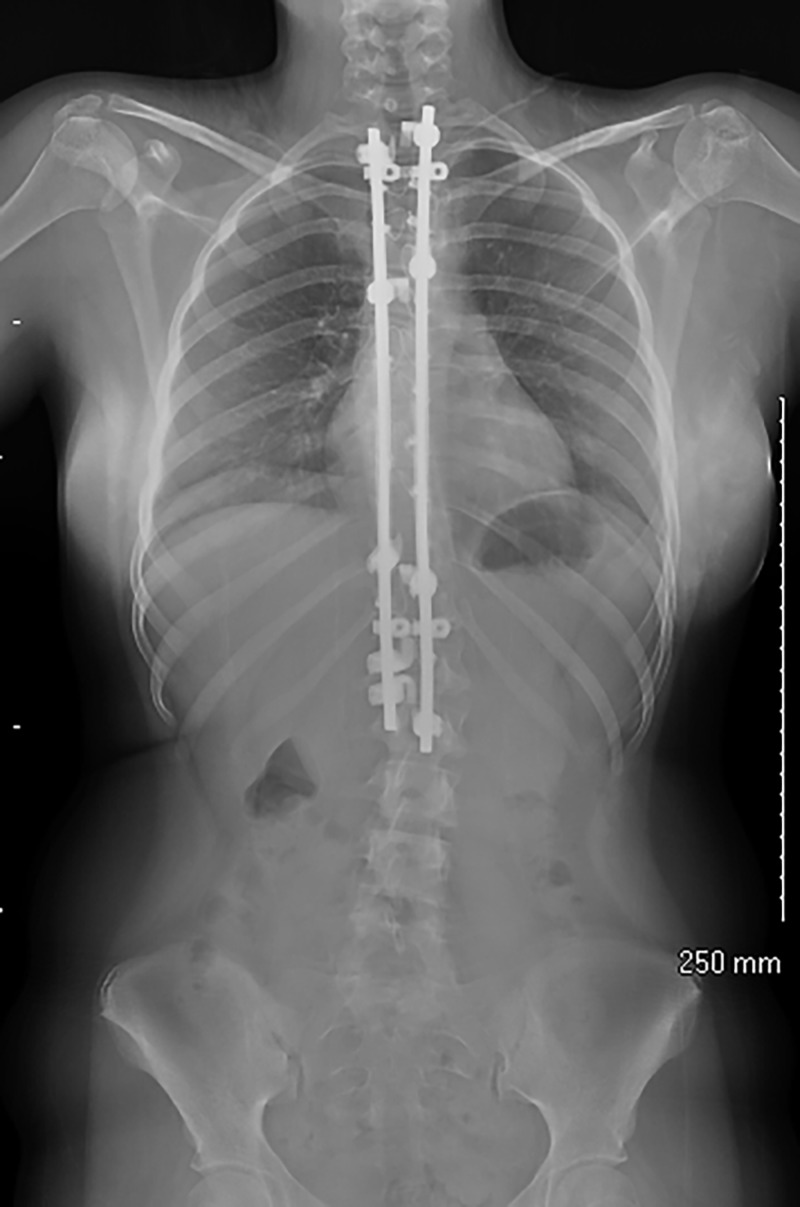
Postoperative AP radiograph demonstrating postoperative correction of scoliosis AP: anteroposterior
